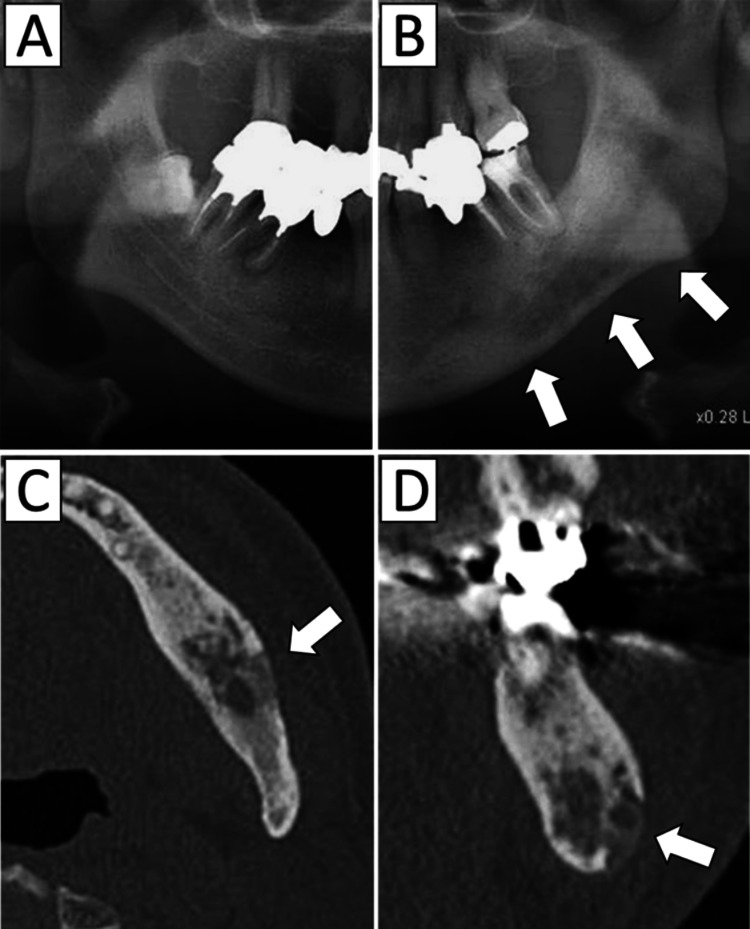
Imaging findings at the first visit to our department A: Panoramic radiograph of the unaffected side B: Panoramic radiograph of the affected side showing diffuse osteolytic changes (arrow) C, D: CT images demonstrating poorly defined osteolytic lesions (arrows) with surrounding osteosclerosis
